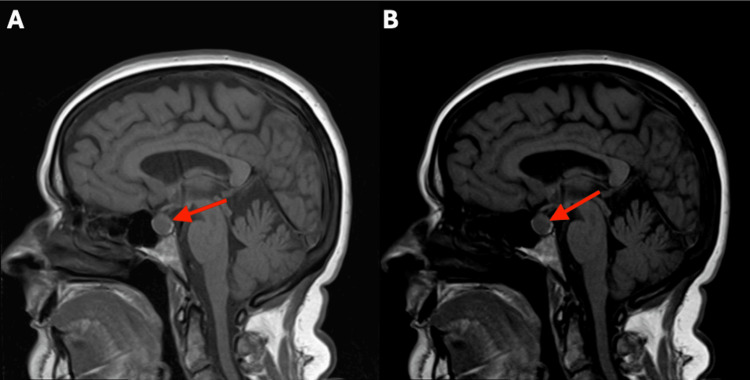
Sagittal T1-weighted (A) and T2-weighted (B) MRIs showing NF-PitNET at the time of presentation The red arrow indicates the NF-PitNET, measuring 1.1 cm × 1.3 cm. NF-PitNET, nonfunctioning pituitary neuroendocrine tumor
